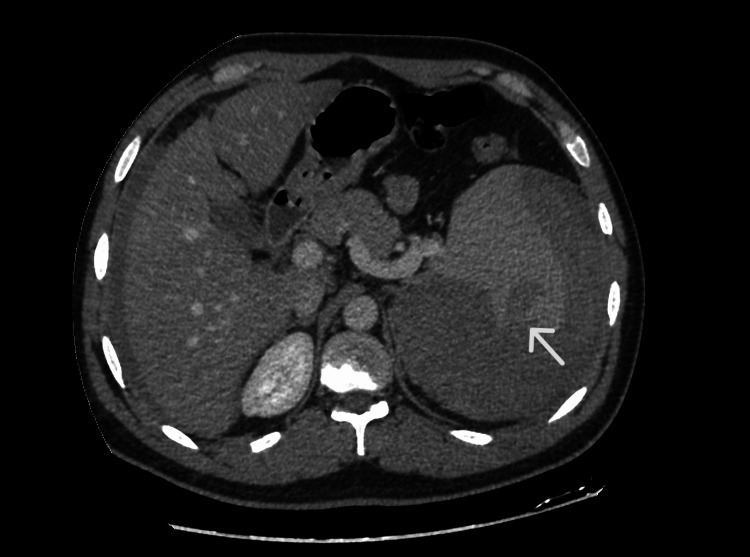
Enhanced CT shows a hypodense lesion through the posterior inferior portion of the spleen with peri-splenic hematoma and abdominal free fluid
